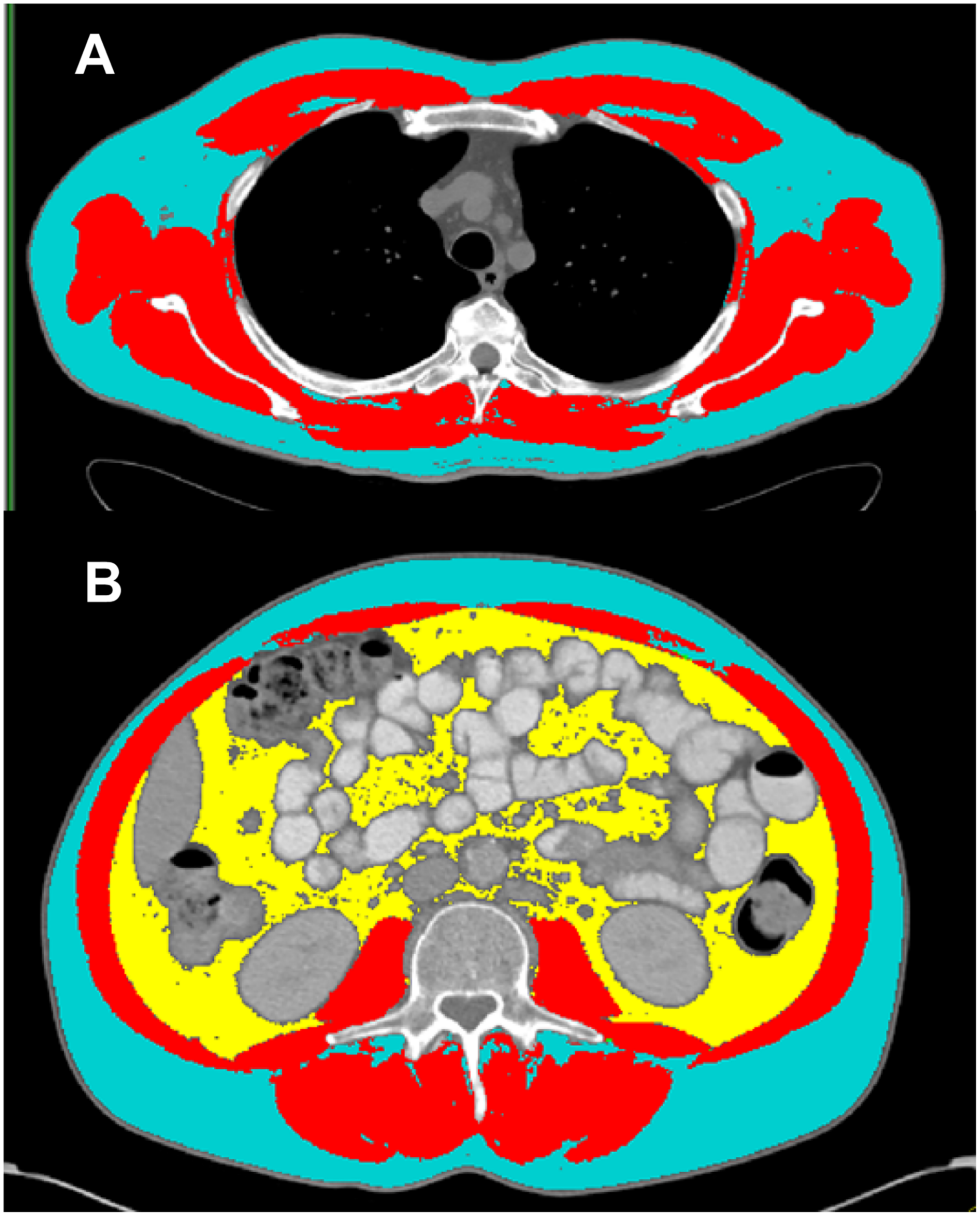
Example thoracic (T4) and lumbar (L3) computed tomography scans with tissue quantification. At the thoracic level (*A*), skeletal muscle is displayed in red, and subcutaneous adipose tissue is displayed in blue. At the lumbar level (*B*), skeletal muscle is displayed in red, subcutaneous adipose tissue in blue, and visceral adipose tissue in yellow.
